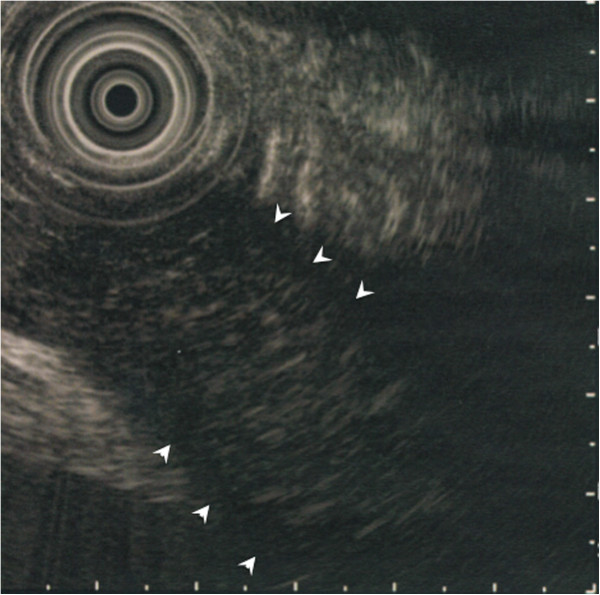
Endoscopic ultrasonographic view showing the low-echoic, enlarged pancreatic tail with a marginal capsule-like rim (arrowhead).
